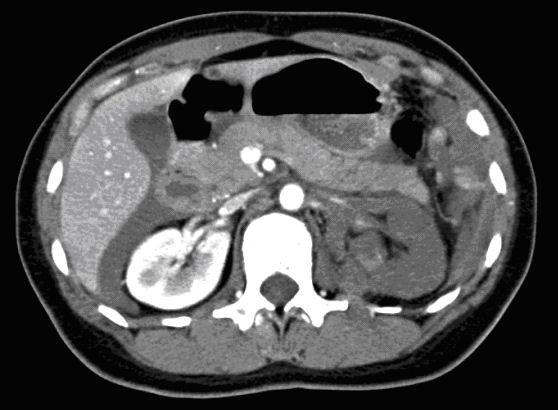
Computed tomography with intravenous contrast: transection of the renal artery without contrast in the left kidney.
